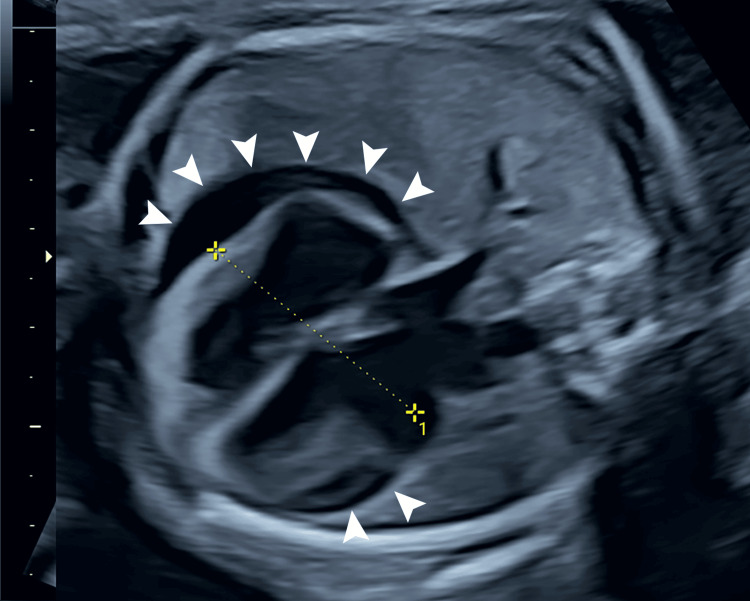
Fetal ultrasonography image at 26 weeks of gestation. The whole circumference of the heart was surrounded by an echo-free space of 7-8 mm (arrowheads), indicating pericardial effusion.
